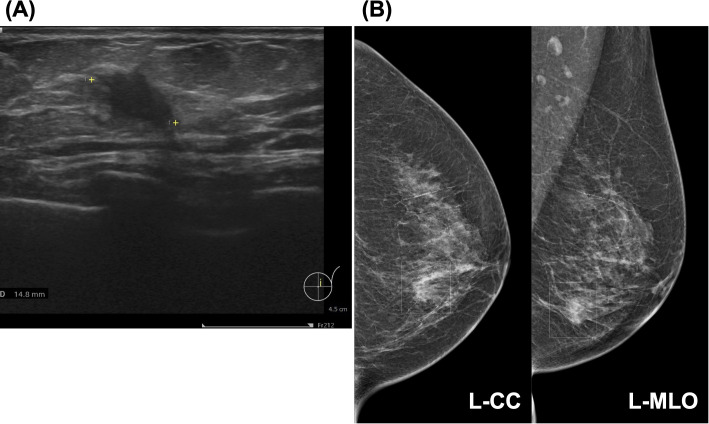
Preoperative breast imaging findings. (A) Breast ultrasonography of the left breast demonstrating an approximately 1.5 cm indistinct hypoechoic subareolar nodule, categorised as BI-RADS 4C. (B) Left breast mammography in craniocaudal (CC) and mediolateral oblique (MLO) views showing a developing asymmetry measuring approximately 1.5 cm in the lower inner quadrant without suspicious calcifications, categorised as BI-RADS 4C.
